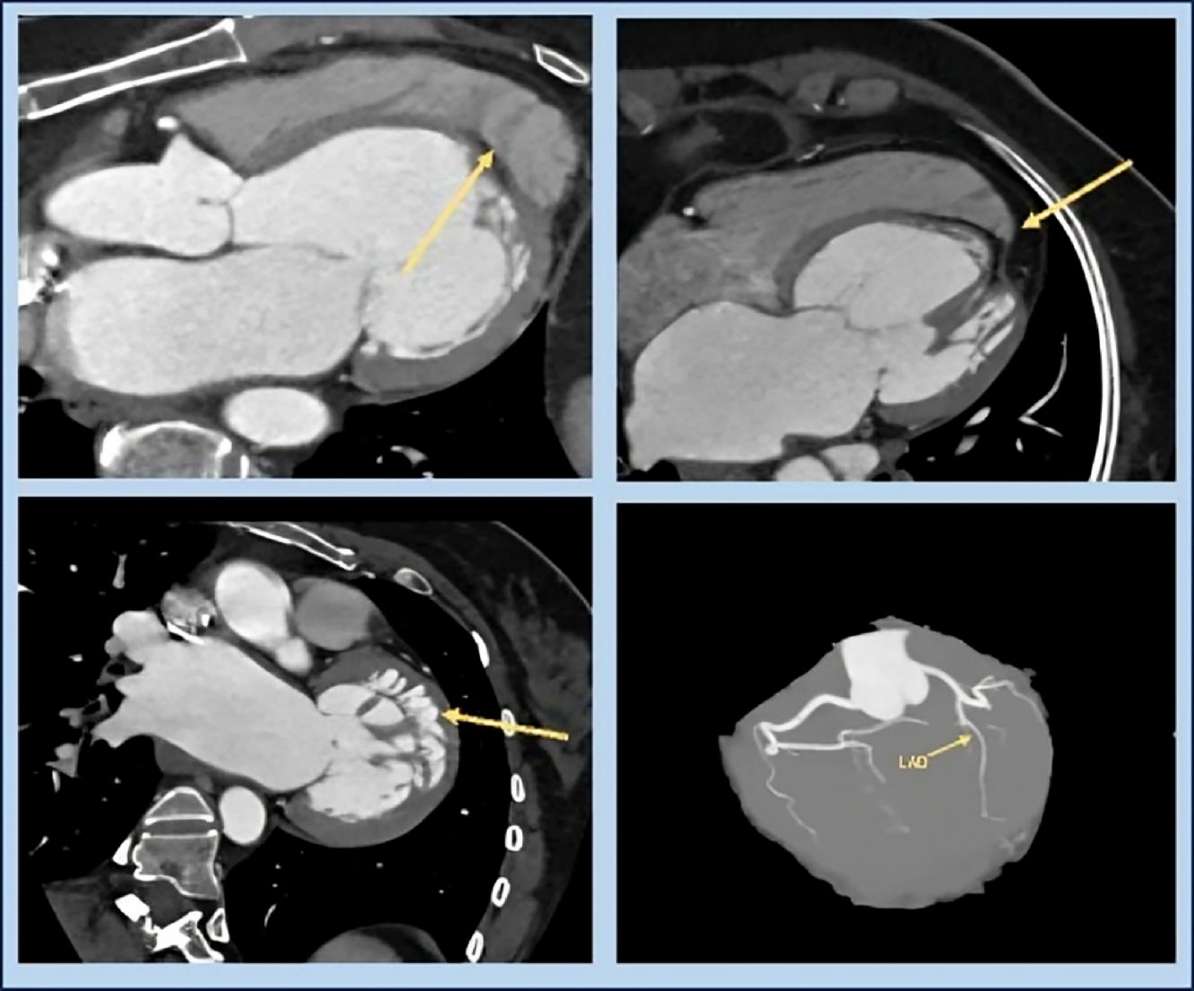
CT coronary angiogram images suggestive of (*A*) wrapping around the right ventricle over the deficient left ventricle apex, (*B*) fatty infiltrating hypoplastic left ventricle apex, (*C*) abnormal origin of papillary muscles and hypertrabeculation, and (*D*) normal coronary arteries with Type I left anterior descending artery.
